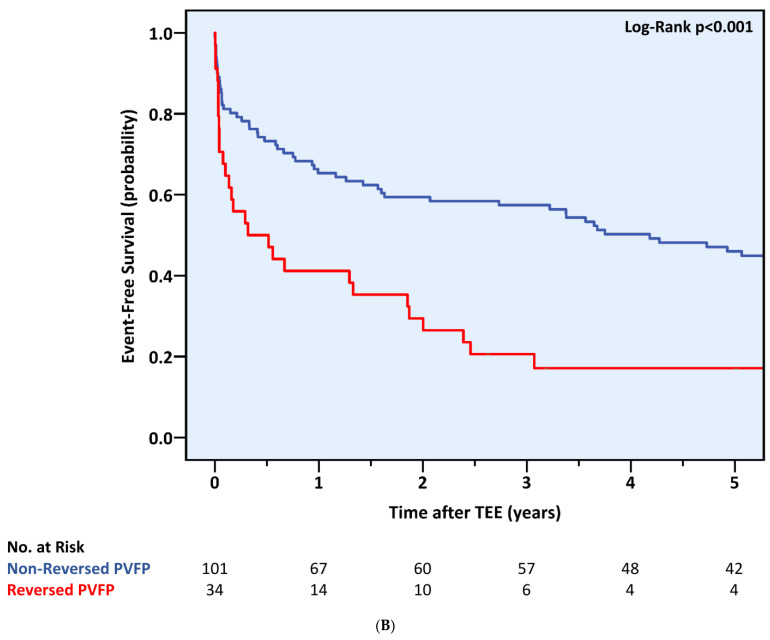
Five-Year Cumulative Incidence of the Combined Outcome of All-Cause Mortality, Mitral Intervention, or New-Onset Atrial Fibrillation According to Pulmonary Venous Flow Pattern at Baseline. PVFP = pulmonary venous flow pattern; TEE = transesophageal echocardiogram. (A) Normal vs. Reversed Pulmonary Venous Flow Pattern. (B) Non-Reversed vs. Reversed Pulmonary Venous Flow Pattern.
